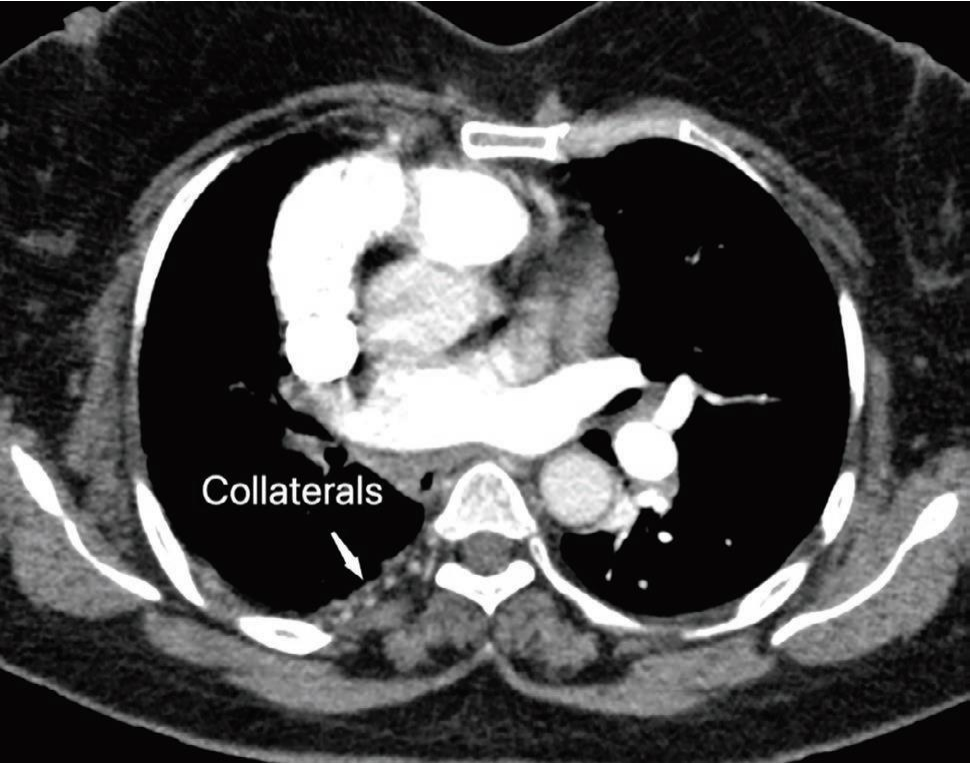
CT pulmonary angiogram phase in axial view shows tortuous, dilated right intercostobronchial arteries supplying the right lung (systemic collateral circulation).
